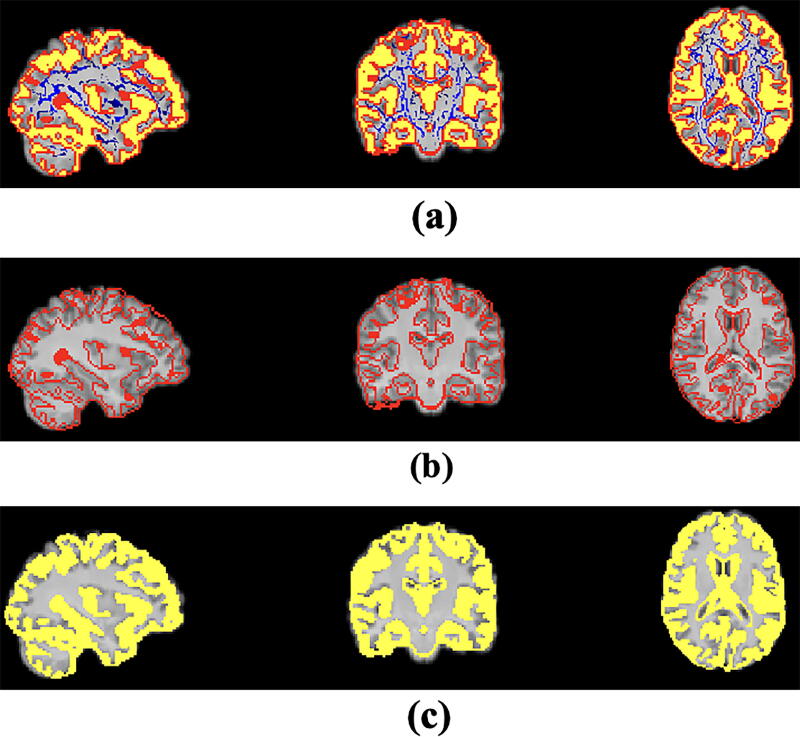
(a) First row is showing all three features of GM general structure, GM surface, and WM skeleton overlapped on skull-stripped T1-weighted image. Individually, (b) the second row is showing the whole brain GM surface (red), and (c) the third row is the general structure (yellow). GM, gray matter; WM, white matter.
